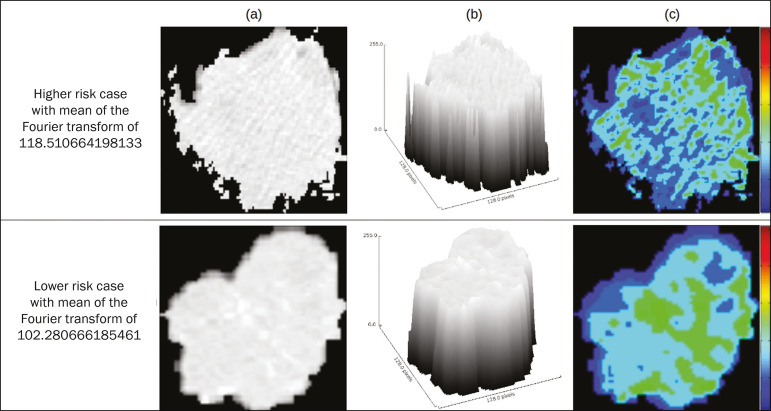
Quantification of tumor heterogeneity in the lesions, stratified by the mean of the Fourier transform. a: Axial CT image, with lung window settings, showing the segmented tumor. b: Three-dimensional distribution of the gray levels. c: Map reflecting the local energy of the tumor with a 5 × 5 pixel window.
